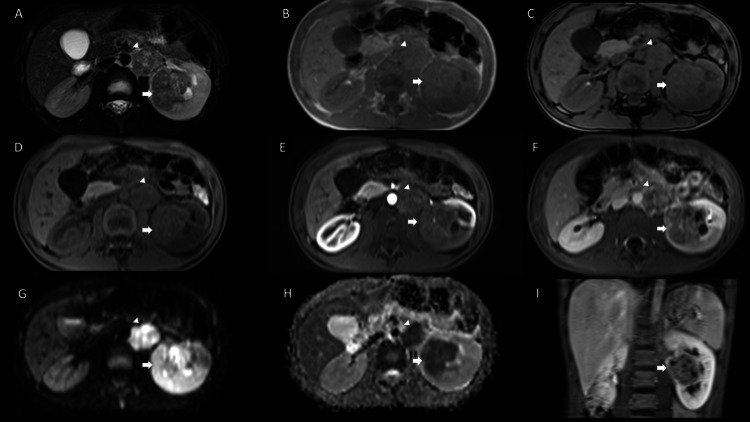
Contrast-enhanced MRI of the abdomen and pelvis. MRI on axial T2-weighted (A), in-phase (B), and out-of-phase T1 sequence (C). T1-weighted imaging with fat saturation (D), post-contrast T1-weighted with fat saturation on arterial phase (E), late phase (F), and coronal image on late phase (I). B800 diffusion sequence (G) and its apparent diffusion coefficient (ADC) map (H). Solid infiltrative lesion centrally located in left kidney, hypointense on T2W (arrow in A), with no out-of-phase signal drop in T1-weighted imaging that indicates any fat component (arrows in C and D); the intermediate signal on T1-weighted imaging (arrow in D); and hypovascular on contrast-enhanced sequences (arrows in E, F, and I). Additionally, retroperitoneal lymphadenopathies are confirmed (arrowhead in A, B, C, D, E, F, G, and H).
